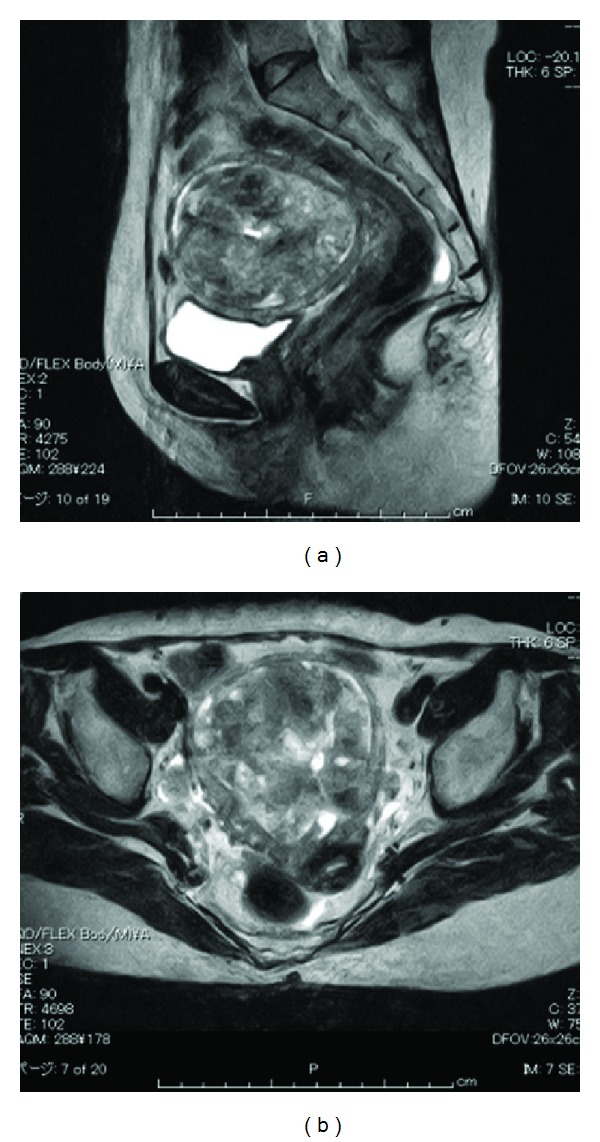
MRI (T_2_-weighted) revealed a heterogeneous solid tumor of 77 × 76 mm.
